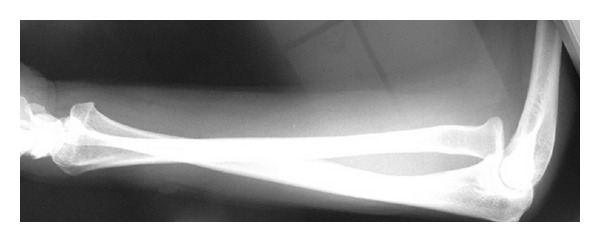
Radiographic findings at the first medical examination. From lateral view, radius head was dislocated anteriorly and distal end of radius was dislocated posteriorly. Radius and ulna were found to have the shape of letter X.
